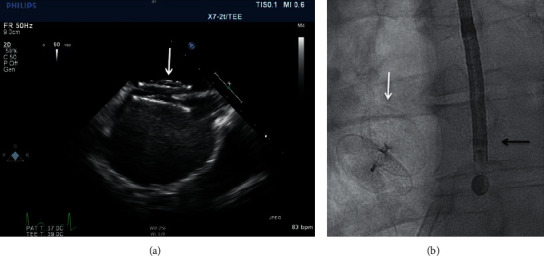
The Occlutech ASD device (white arrow) using the microtransesophageal echocardiography (micro-TEE) probe (a) and fluoroscopy (b). The black arrow indicates the micro-TEE probe during fluoroscopy.
